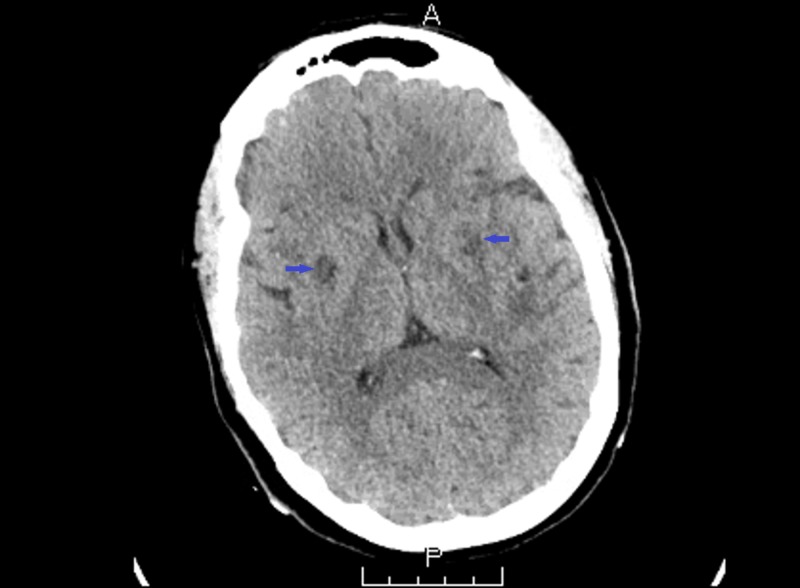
Computed tomography (CT) head non-contrast showing areas of diminished density in basal ganglia bilaterally (highlighted by blue arrows).
